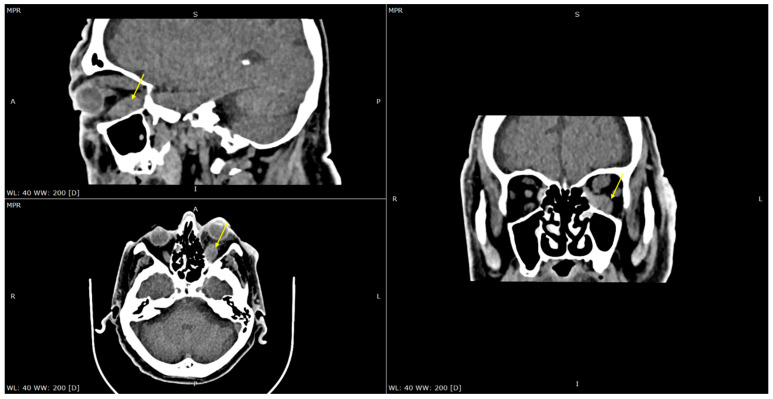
Multiplanar reconstruction of computed tomography. Generalized thickening and edema of all the extraocular muscles, mostly evident in the lower rectus muscle (arrow).
